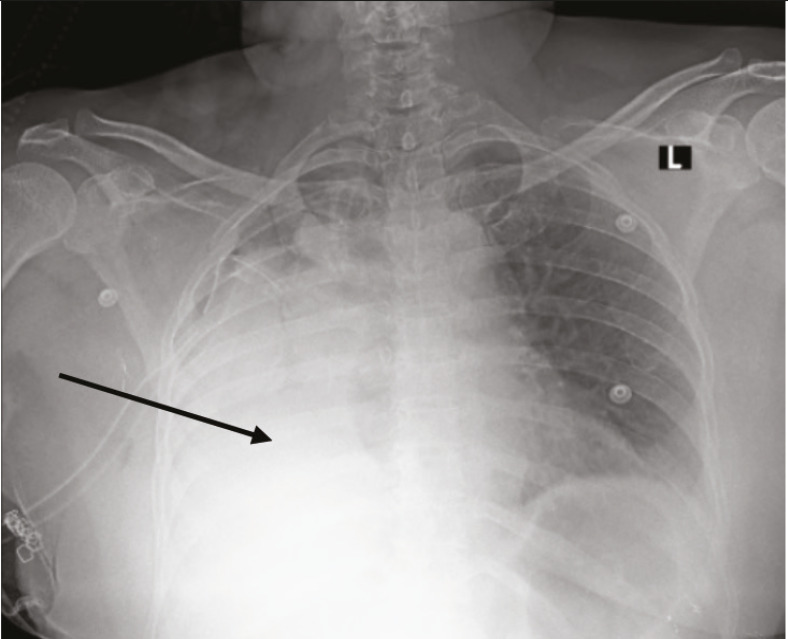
Postoperative chest radiograph showing total collapse of the right hemithorax. L: left.
